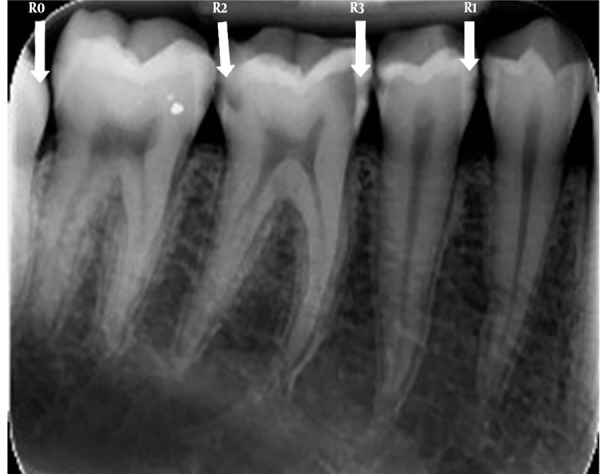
Classification of caries according to the depth of caries in radiography. Sound (R0), restricted to the enamel (R1), reaching the dentinoenamel junction, reaching the outer half of the dentin (R2) and the inner half of the dentin (R3)
